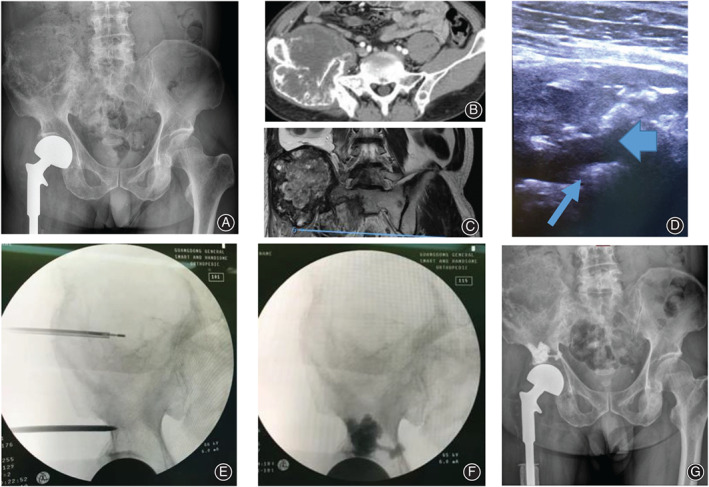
(A) A 59‐year‐old patient with lung cancer who was previously treated with hip prosthesis reconstruction for multiple hip metastases, including metastasis to the right acetabulum. (B, C) CT and MRI show osteolytic metastases with a large soft tissue mass in the right iliac bone. (D) This case underwent percutaneous microwave ablation guided with X‐ray combined with ultrasound. The ablation zone during microwave ablation (thin arrow), an osteolytic zone with soft tissue mass (thick arrow), which were shown on ultrasound. (E) The microwave probe guided by fluoroscopy, With the aid of ultrasound, the microwave needle was located in the lesion and adjusetd the Angle and depth of the needle gradually. (F) After the ablation, cementoplasty was performed guided with fluoroscopy. (G) The postoperative fluoroscopy showed no leakage of bone cement.
